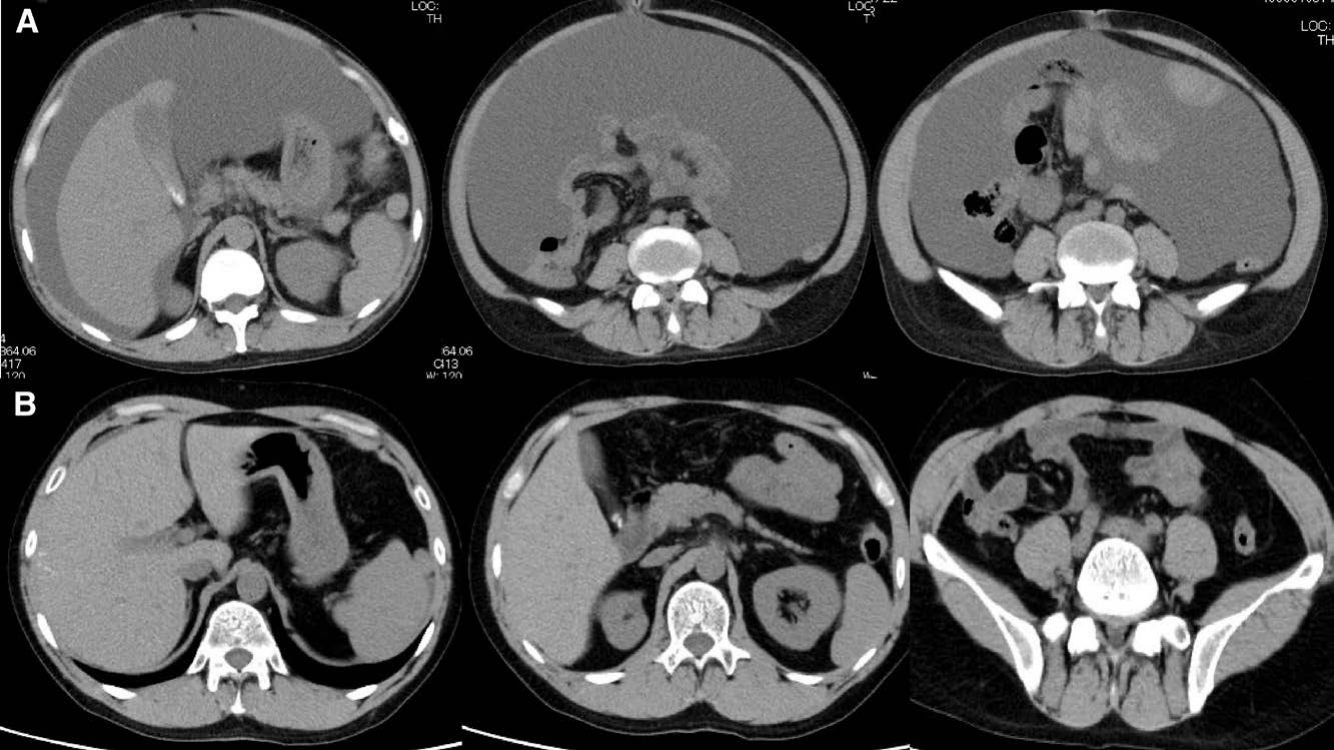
Abdominal computed tomography image. (A) A large number of ascites is observed before ruxolitinib. (B) Resolution of ascites after treatment with ruxolitinib.
